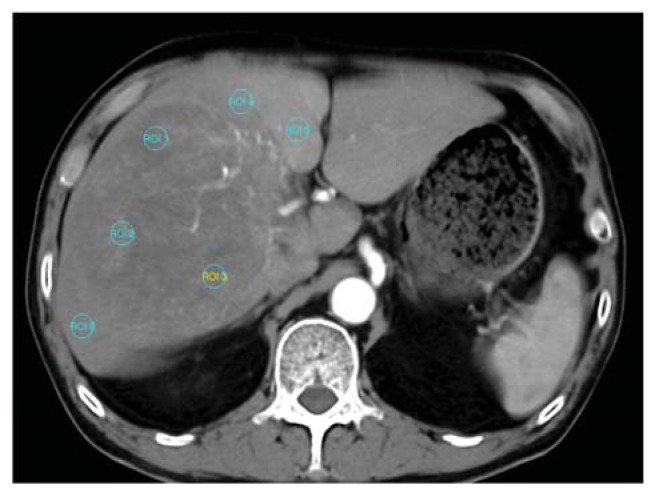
Six similar-sized region of interest (ROI) areas (1 mm^2^) are shown for measuring the CT perfusion BF of target HCC (ROI 1–3) and the adjacent non-tumor liver parenchyma (ROI 4–6).
